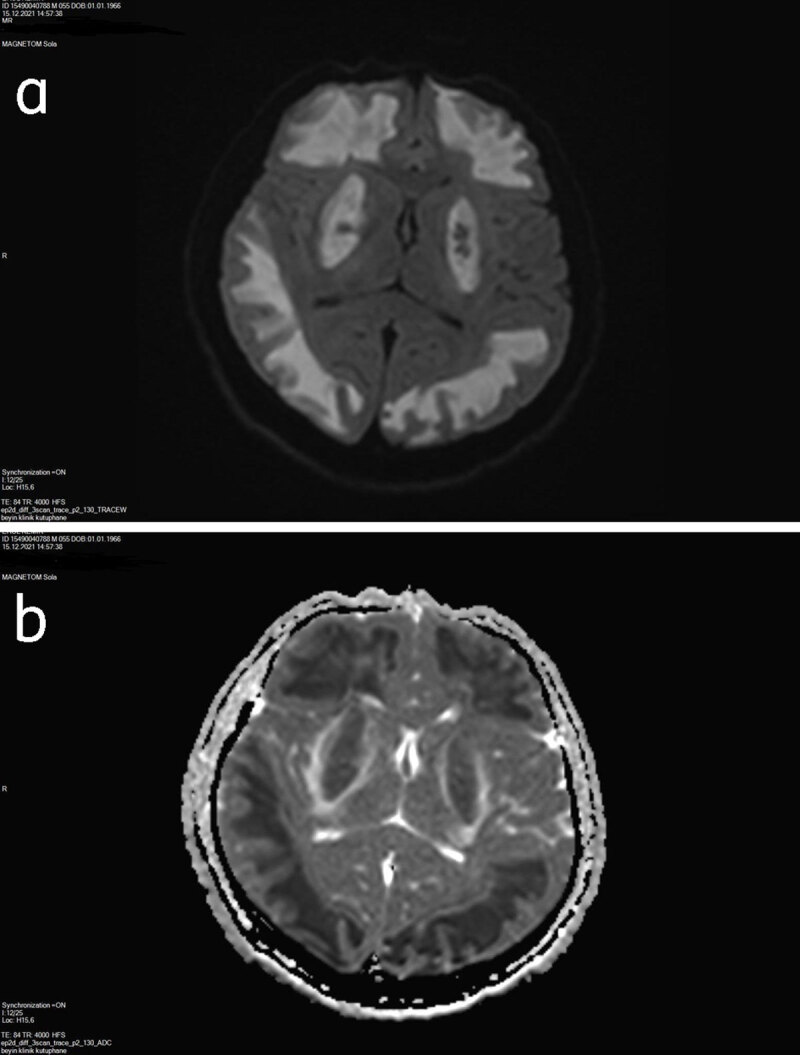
a DWI reveals increased signal intensity on both putaminal and cerebral subcortical white matter regions. b ADC map shows decreased signal intensity on corresponding brain regions indicating restricted diffusion due to cytotoxic edema.
